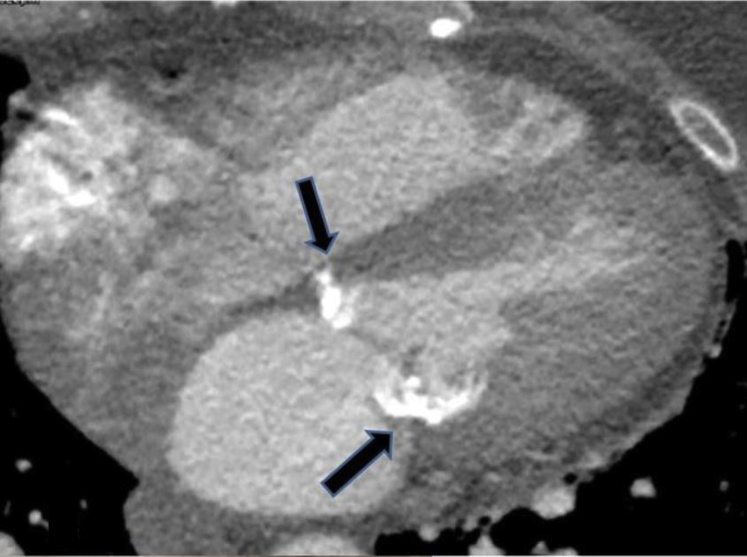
Mitral annular calcification with deep ventricular extension (*arrows*) can be identified on preoperative computed tomography.
